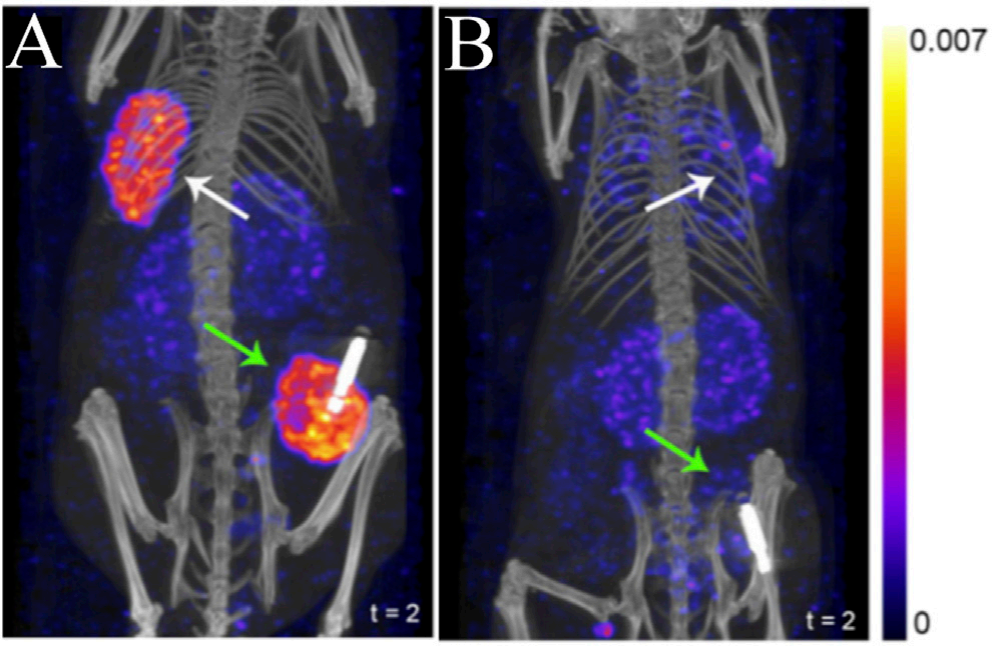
SPECT/CT imaging using ^111^In-JMV4168 in orthotopic (green arrows) and subcutaneous (white arrows) tumors of T47D (A) and MCF7 (B) xenografts at 4 h post-injection of ^111^In-JMV4168 (Dalm et al., 2015). The general structure of ^111^In-JMV4168 was shown in Figure 2D.
